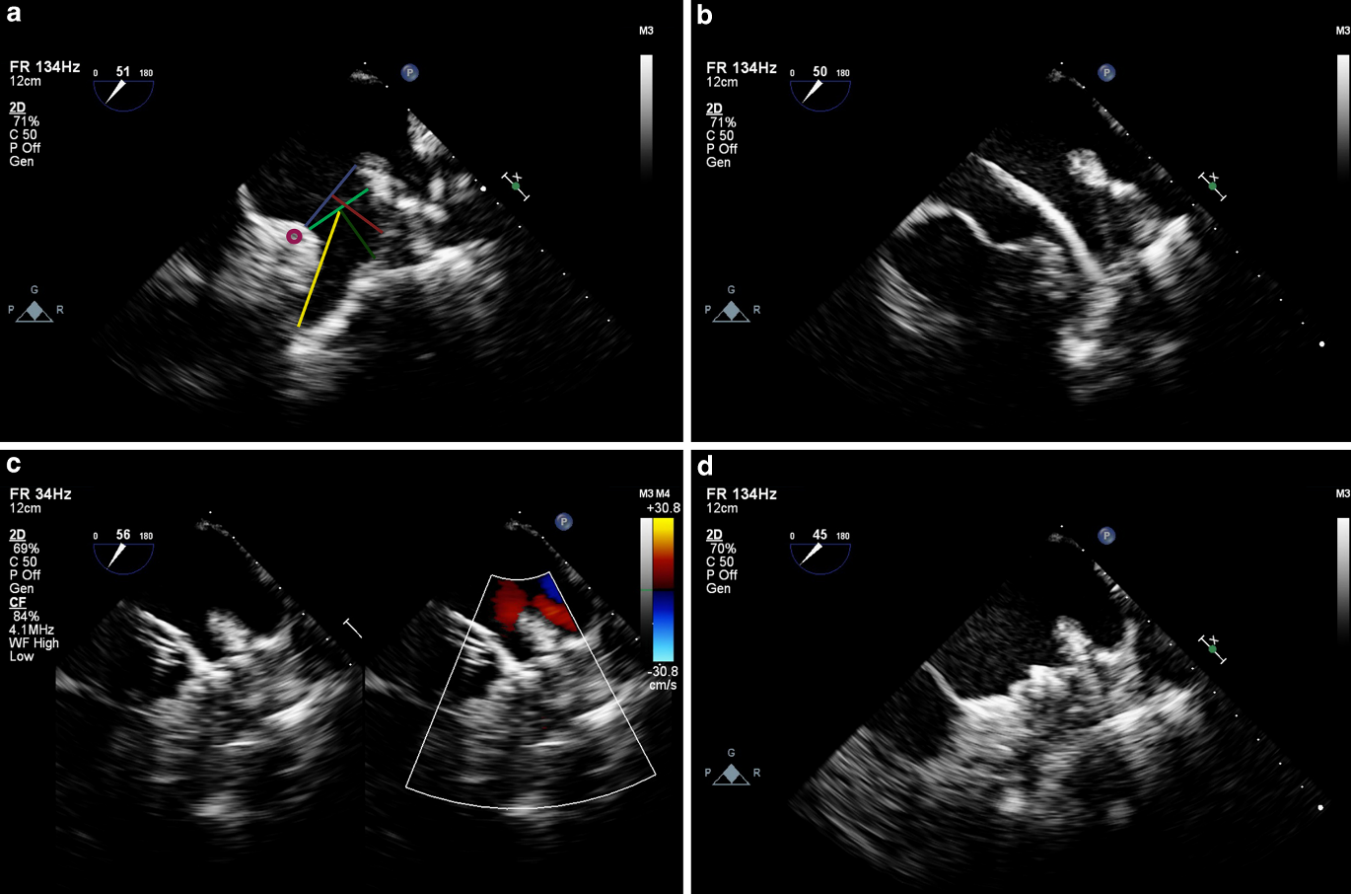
Microtransoesophageal echocardiographic images during left atrial appendage closure. Microtransoesophageal echocardiography during left atrial appendage (LAA) closure. a The LAA is shown at 51 degrees, and the dimensions routinely measured for LAA closure are drawn: ostium (blue), landing zone (light green), depth of ostium (red), depth of landing zone (dark green), and the total depth (yellow). b A Watchman device is advanced in the LAA. c The Watchman device is deployed and colour compare mode shows no residual flow. d The Watchman device is released
